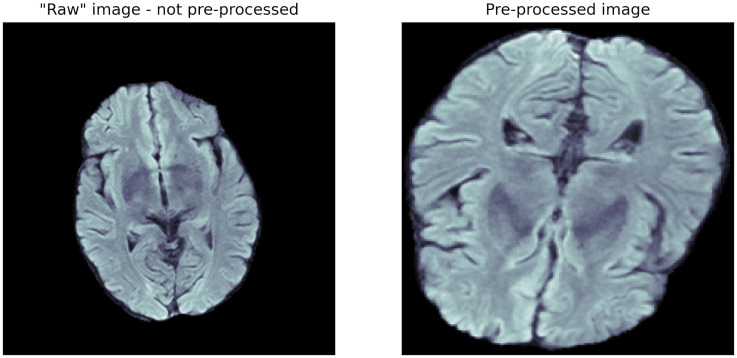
Comparison between non-pre-processed and pre-processed scans. The left image is a brain scan under the FLAIR MRI sequence, and we did not apply any pre-processing on this image. The right image is the same after the pre-processing steps: re-orientation, cropping to center the image, resizing, and standardization.
